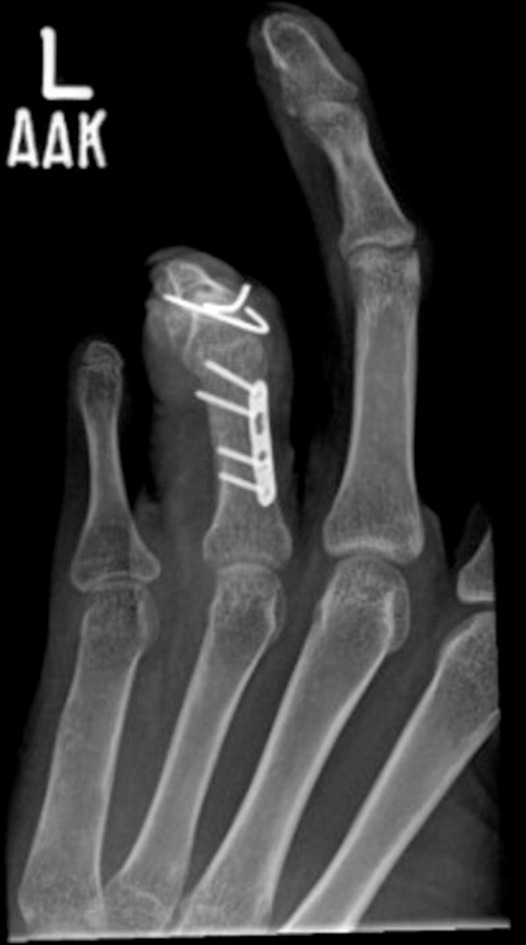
Post-operative hand radiograph. Post-operative x-ray *showing* separation of third and fourth fingers of left hand.
